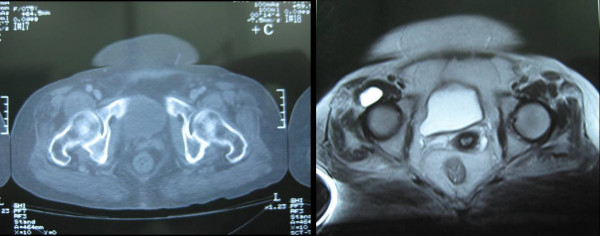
Computer tomography scan and magnetic resonance imaging of the right hip region revealed a cystic lesion of 2.6 × 1.4 cm in diameter, displacing the femoral nerve as well as the femoral vein and artery.
